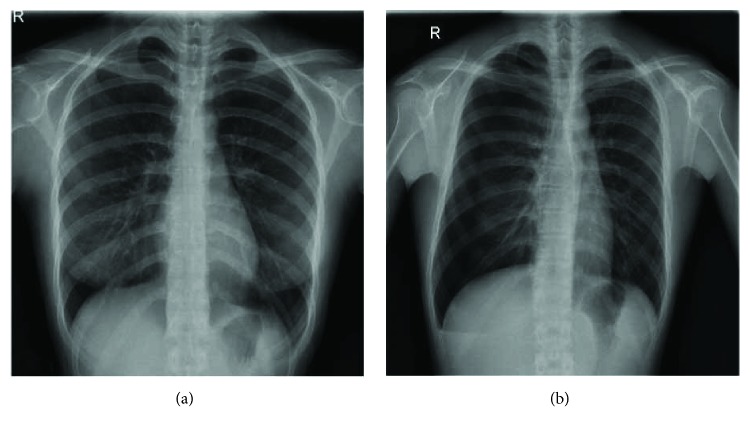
Chest radiographs of (a) normal and (b) pneumothorax.
